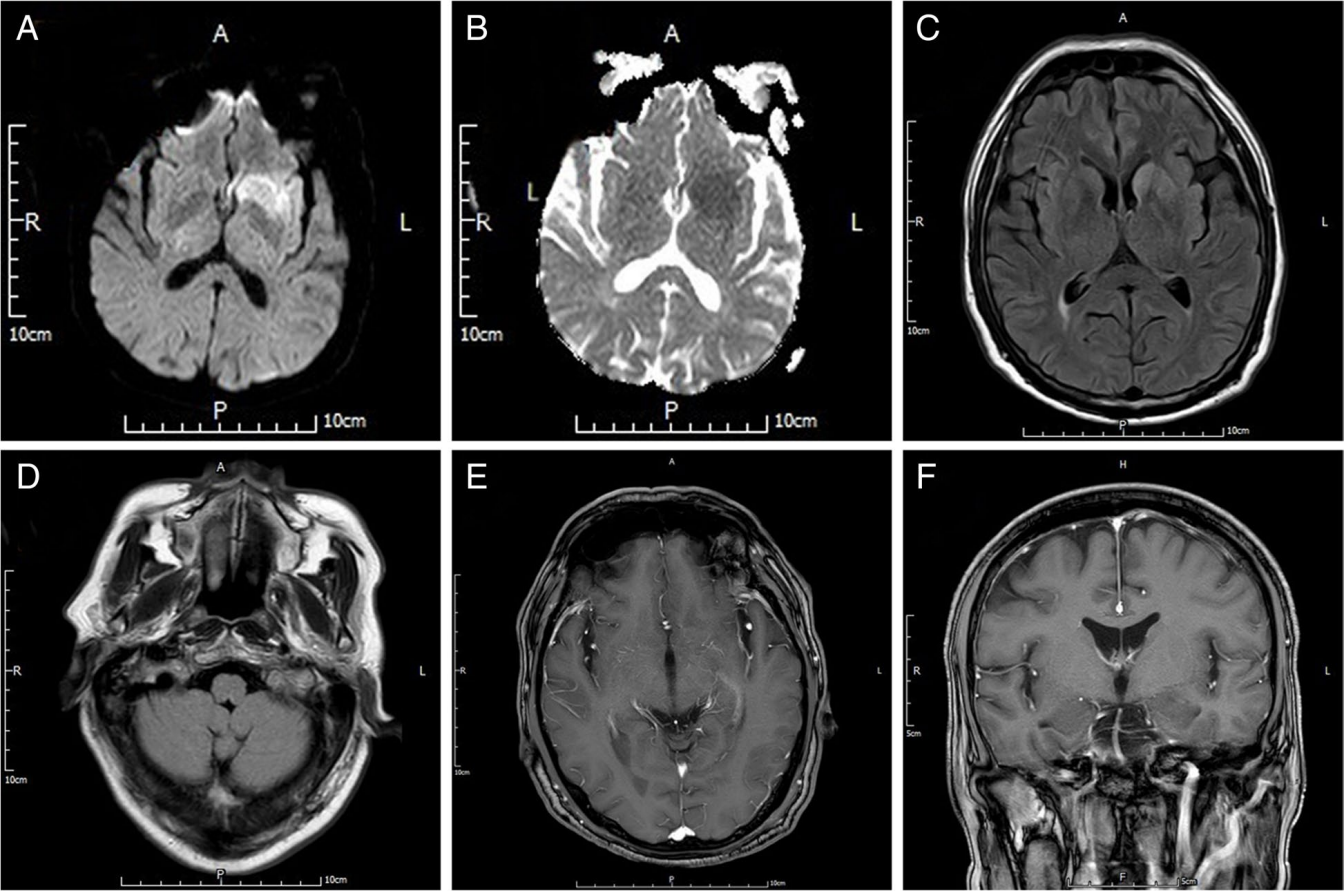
MRI sequences on the first admission. These images demonstrated irregular lesions in the left caudate nucleus, putamen and insula with restricted diffusion (A-C). Medulla oblongata was not affected (D). There was no obvious enhancement of the lesion on contrast-enhanced MRI (E, F). (A) DWI, (B) ADC, (C and D) FLAIR, and (E and F) T1 postcontrast
